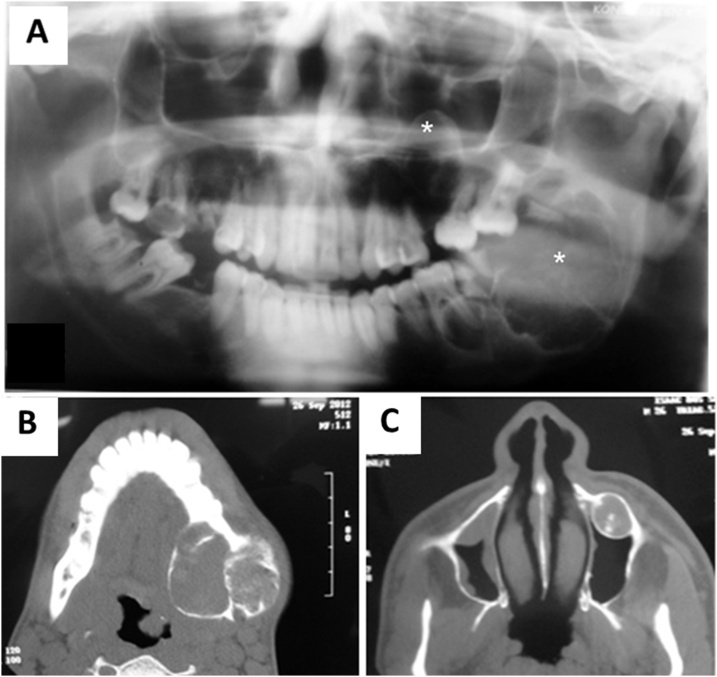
Panoramic radiograph. (A) Note two well-delimited osteolytic lesions, unilocular in the left maxilla and multilocular in the mandible (asterisk). Computed tomography. (B) Note larger diameter lesion involved the mandibular body, angle and ramus, sometimes with a characteristic of hyperdensity, which suggests areas of mineralization. (C) Note lesion in the maxilla associated with hyperdense foci and extended to the maxillary sinus.
